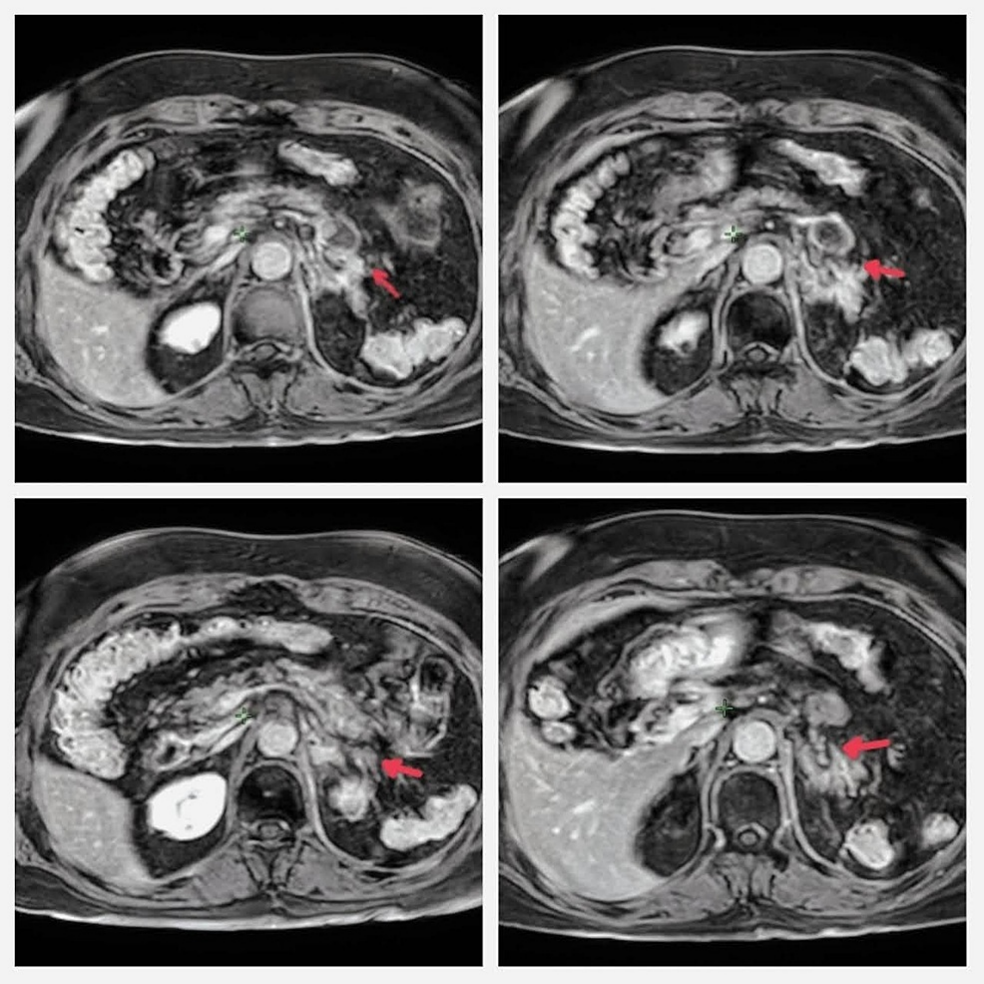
Pre-treatment abdominal MRI In the left suprarenal bed, a hyperintense mass can be identified (arrow) that distorts the hypointensity of the peritoneal fat and extends to the front of the upper pole of the left kidney.
